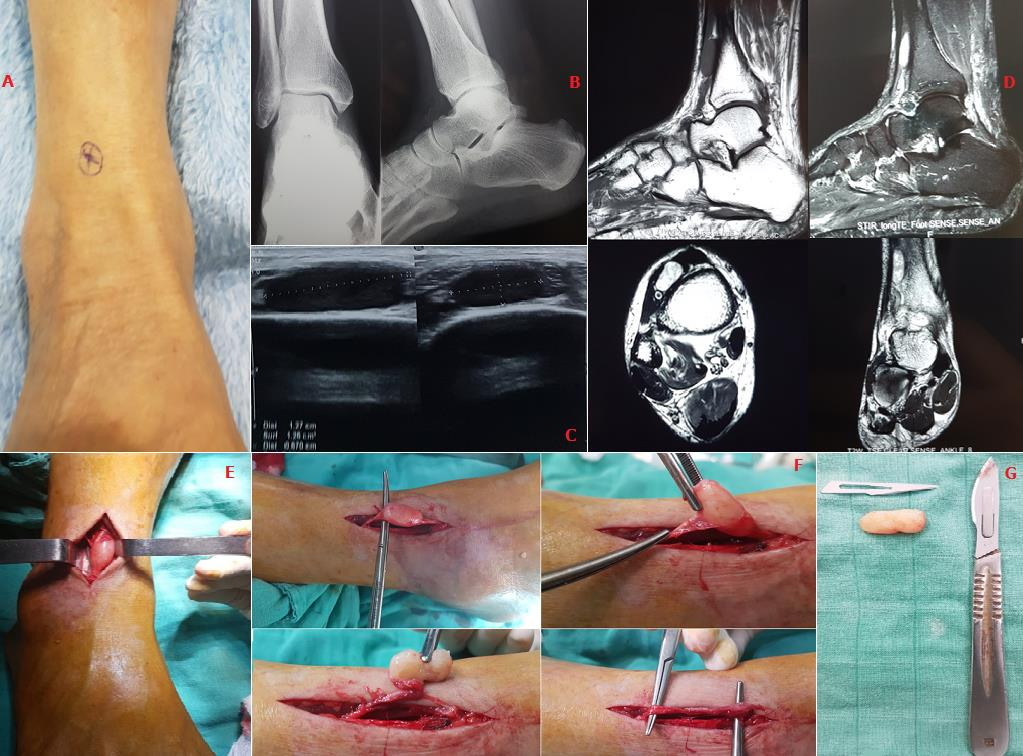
(A) image clinique qui montre la localisation du point douloureux chez notre patient (signe de tinel positif); (B) radiographie standard éliminant une cause ostéoarticulaire sous-jacente; (C) échographie: formation tissulaire oblongue homogène bien limitée; (D) aspect de la tumeur sur l’IRM: masse tissulaire bien limitée, fusifome, en hyposignal en T1, en hypersignal T2 et STIR, se réhaussant faiblement après injection de godolinium; (E) image péropératoire: localisation de la tumeur; (F) images peropératoires: les étapes de dissection de la tumeur autour du nerf (énucléation); (G) aspect final de la pièce tumorale
